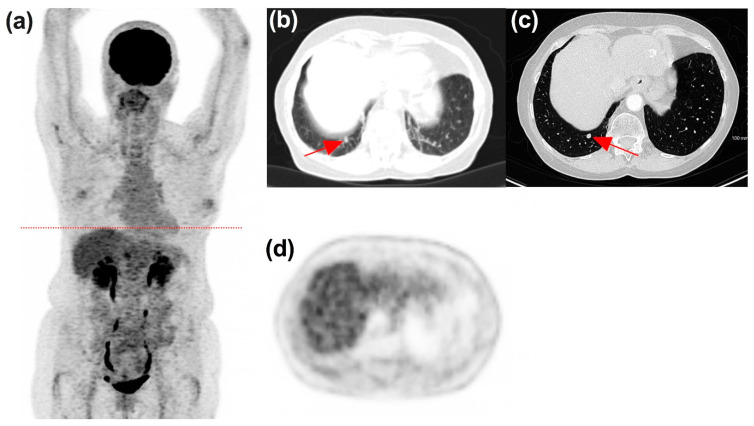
60-year-old female patient diagnosed with stage IV choroidal melanoma (BRAF-) treated with nivolumab. ceCT— (a), maximum intensity view; (b), low-dose CT from the PET/CT scan transverse slice; (c) diagnostic CT transverse slice; (d) PET transverse slice depicted progression of one pulmonary nodule after 3 cycles of treatment, while ^18^F-FDG PET/CT determined stable disease. Note the nodule overlooked on low-dose CT (red arrow, (b)) and not ^18^F-FDG avid (d), and well seen on diagnostic CT (red arrow, (c)).
